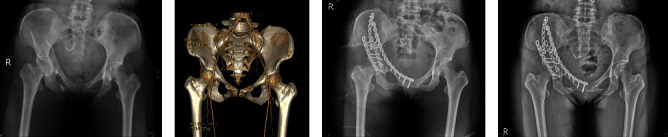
(a) Radiograph of a 47-year-old woman with acetabular fractures and central hip dislocation (AO/OTA 62B3.2). (b) Three-dimensional CT reconstruction. (c) Postoperative radiograph of using dual approaches. (d) Radiograph at final follow-up 405 days after surgery.
